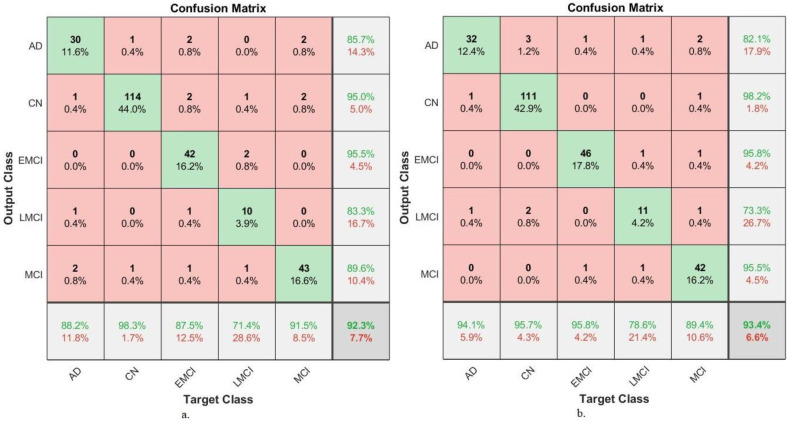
Confusion matrix for generalization performance of FFNN for MRI image analysis of an ADNI dataset based on fused features between (a) GoogLeNet and handcrafted and (b) DenseNet-121 and handcrafted.
